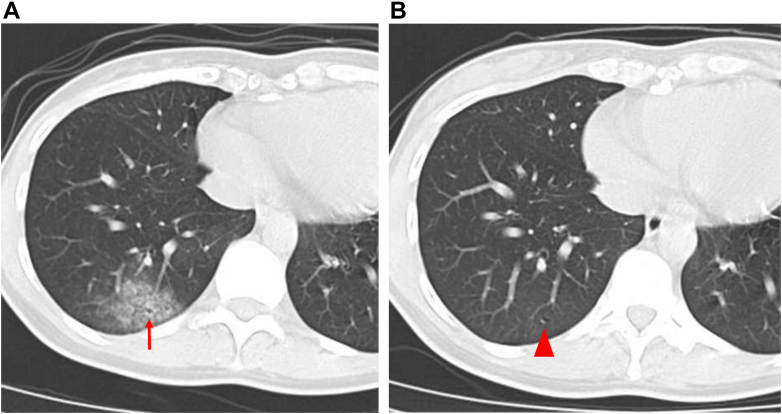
A, B, During menstruation and the midportion of the menstrual cycle CT images. A, CT scan conducted during menstruation demonstrates ground-glass opacity in the right lower lobe surrounding a cystic lesion (arrow). B, CT scan during the midportion of the menstrual cycle shows no ground-glass opacity but the remained dilated distal bronchus (arrowhead).
